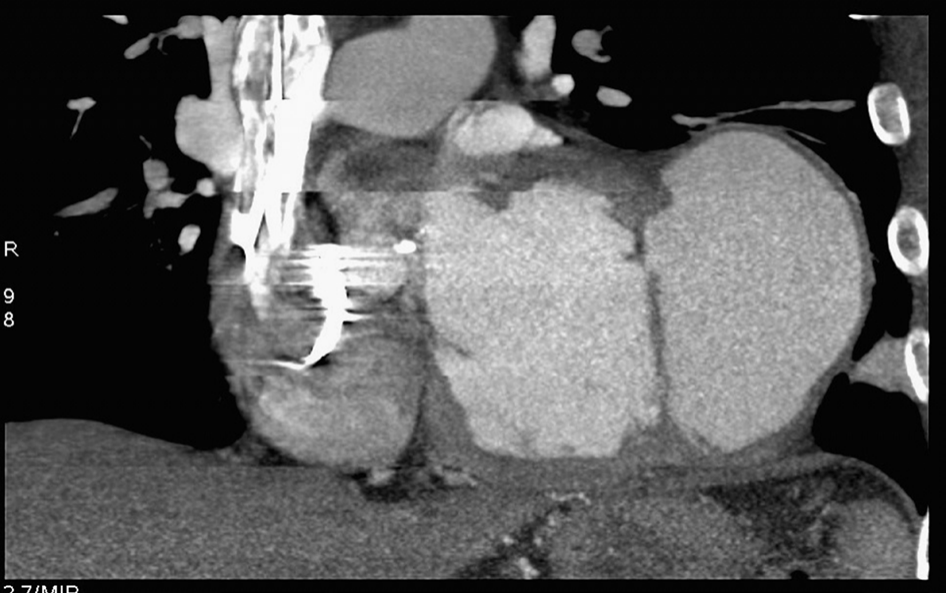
Preoperative CT scan showing the left ventricular pseudoaneurysm
